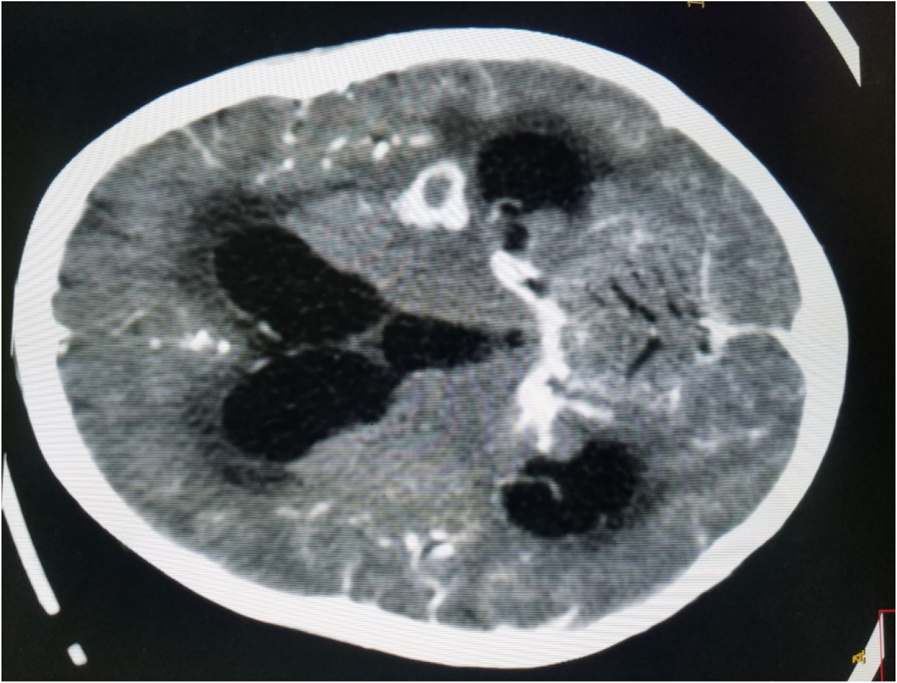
Brain computed tomographic scan showing ring-enhancing lesions with intense basal cisterns exudate
